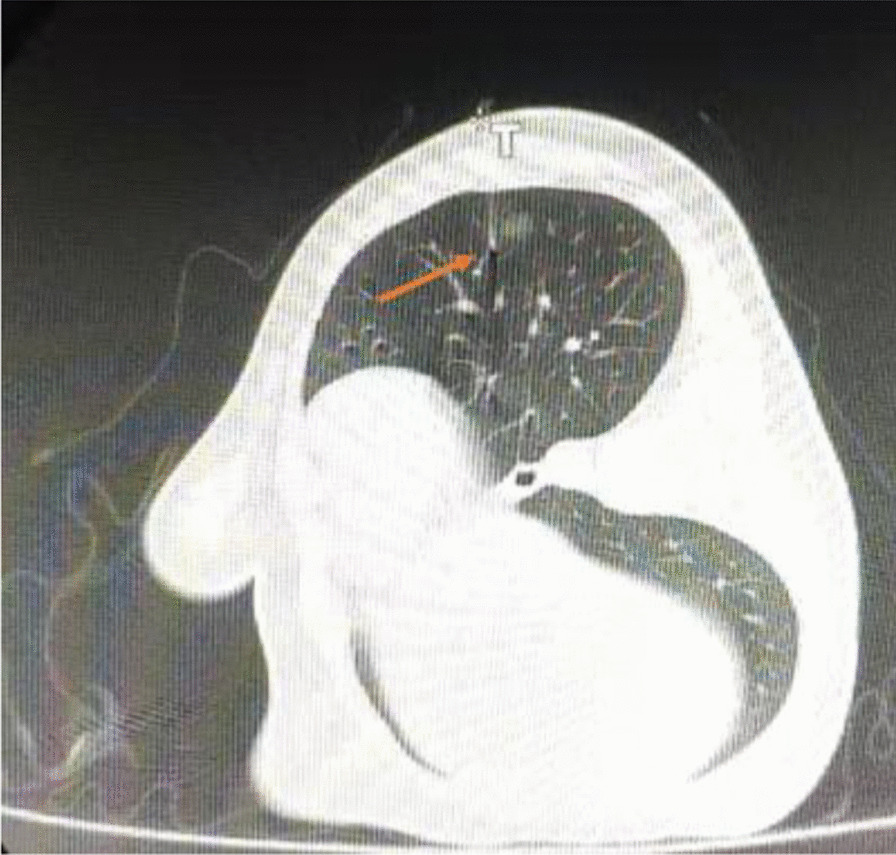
Computed tomography was performed again to ensure the wire location. The hook-shaped wire tip (arrow) was secured at a distance of 1.0 cm from the nodule
